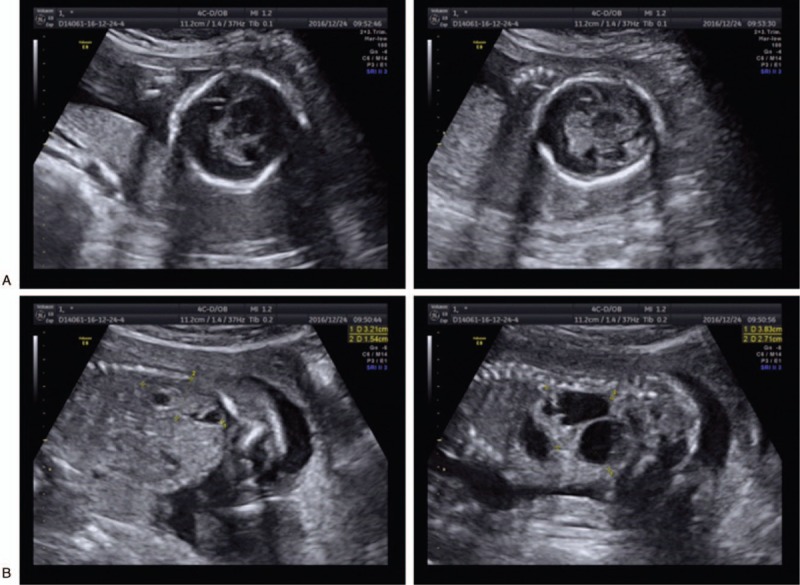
Prenatal ultrasound at 20 weeks of gestation shows the fetus with single ventricle in intracalvarium, thalmus partially fused (A) and multicystic dysplastic kidneys (B).
